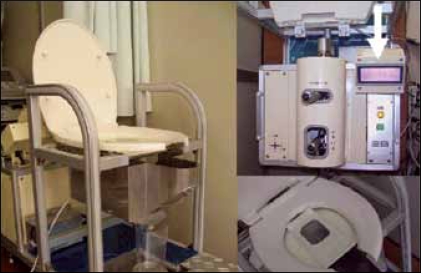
A specially devised perineal ultrasound probe robotic holder. Subjects sat on an uroflow micturition chair. The ultrasound probe was covered with solid echogel and advanced via remote control to achieve gentle contact with the patient's perineal skin, manipulating levers using the position indicator. Arrows indicate the position indicator.
